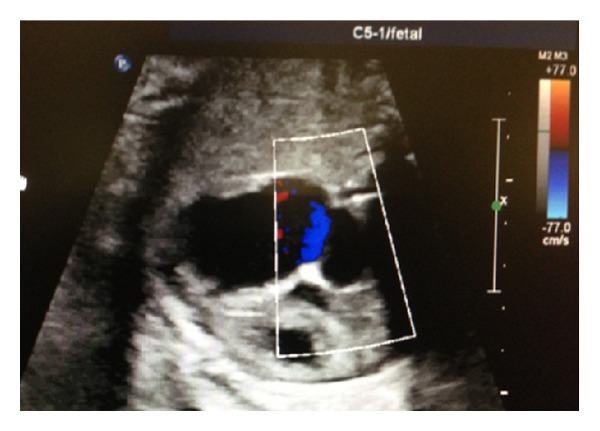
Fetal echocardiography demonstrating right atrial and ventricular enlargement.
